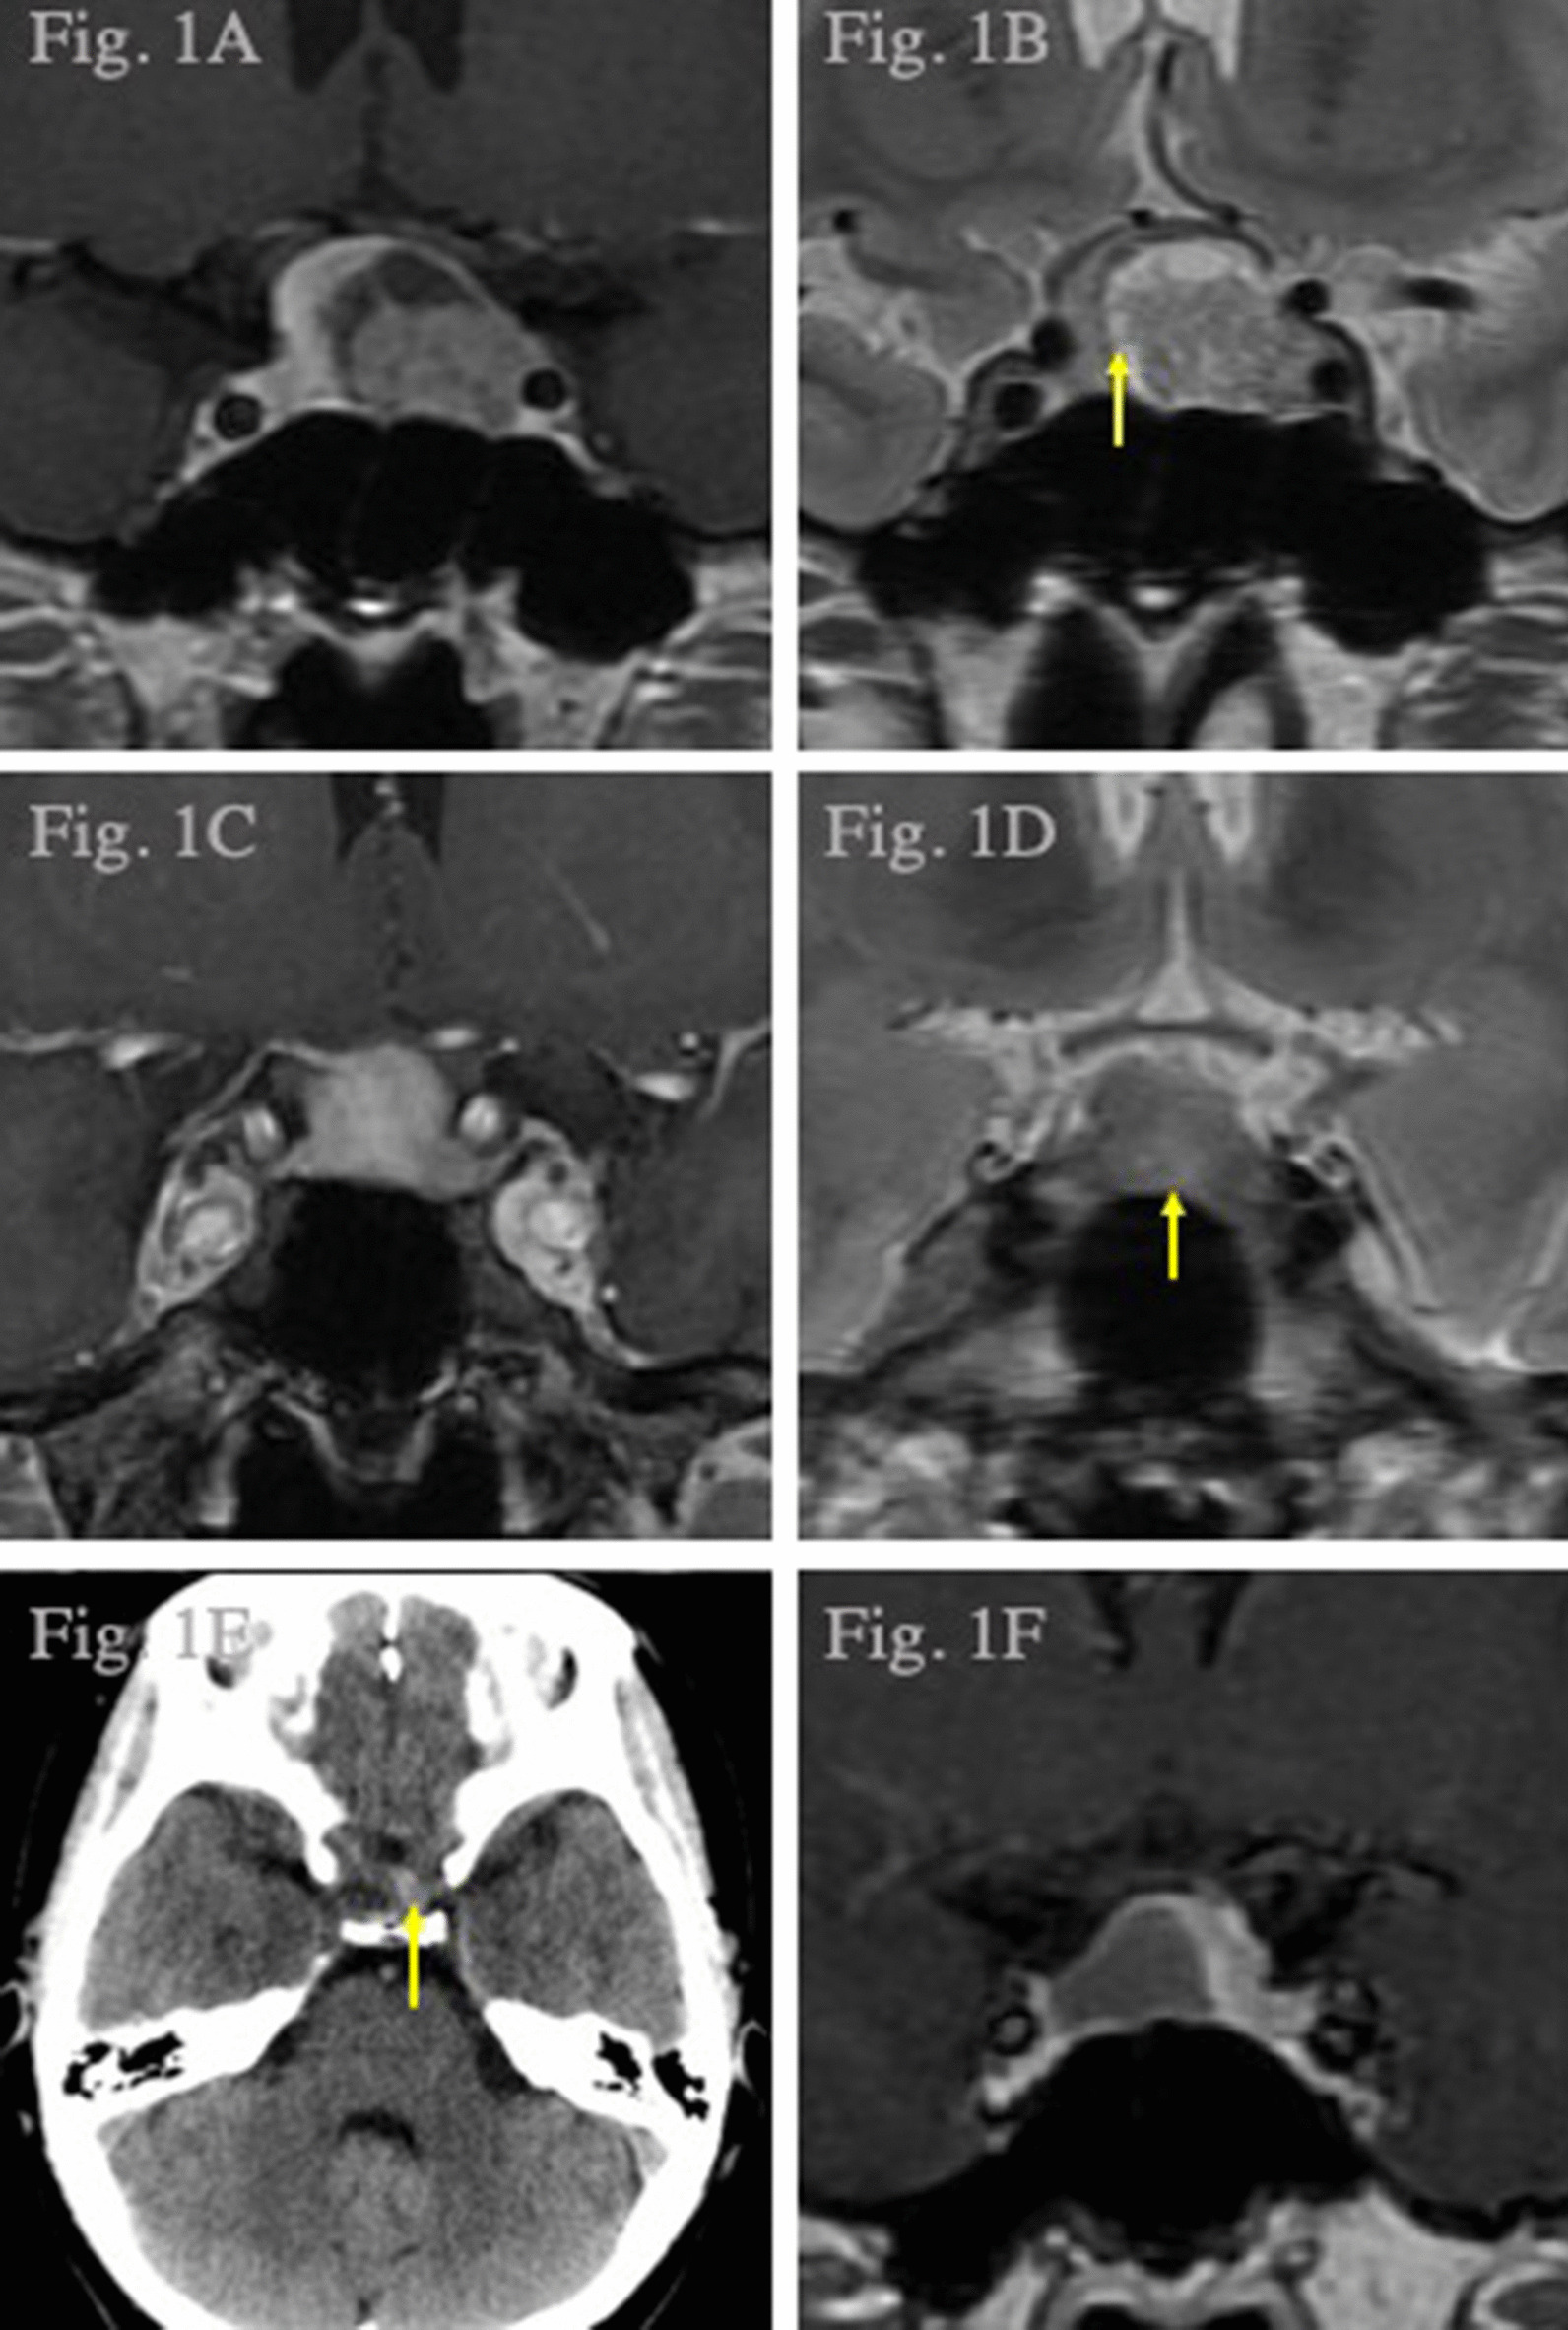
Coronal T1-weighted MR images of the head with contrast medium (case 1, a). Coronal T2-weighted head MR image; yellow arrow shows the hematoma in the tumor (case 1, b). Coronal T1-weighted head MR images with contrast medium (case 2, c). Coronal T2-weighted head MR image with the yellow arrow showing the hematoma in the tumor (case 2, d). Computed tomography scan of the head with the yellow arrow showing hemorrhage in the sella turcica (case 3, e). Coronal T1-weighted head MR images with contrast medium (case 3, f) showing a large sellar tumor that was compressing the optic chiasm anteriorly
